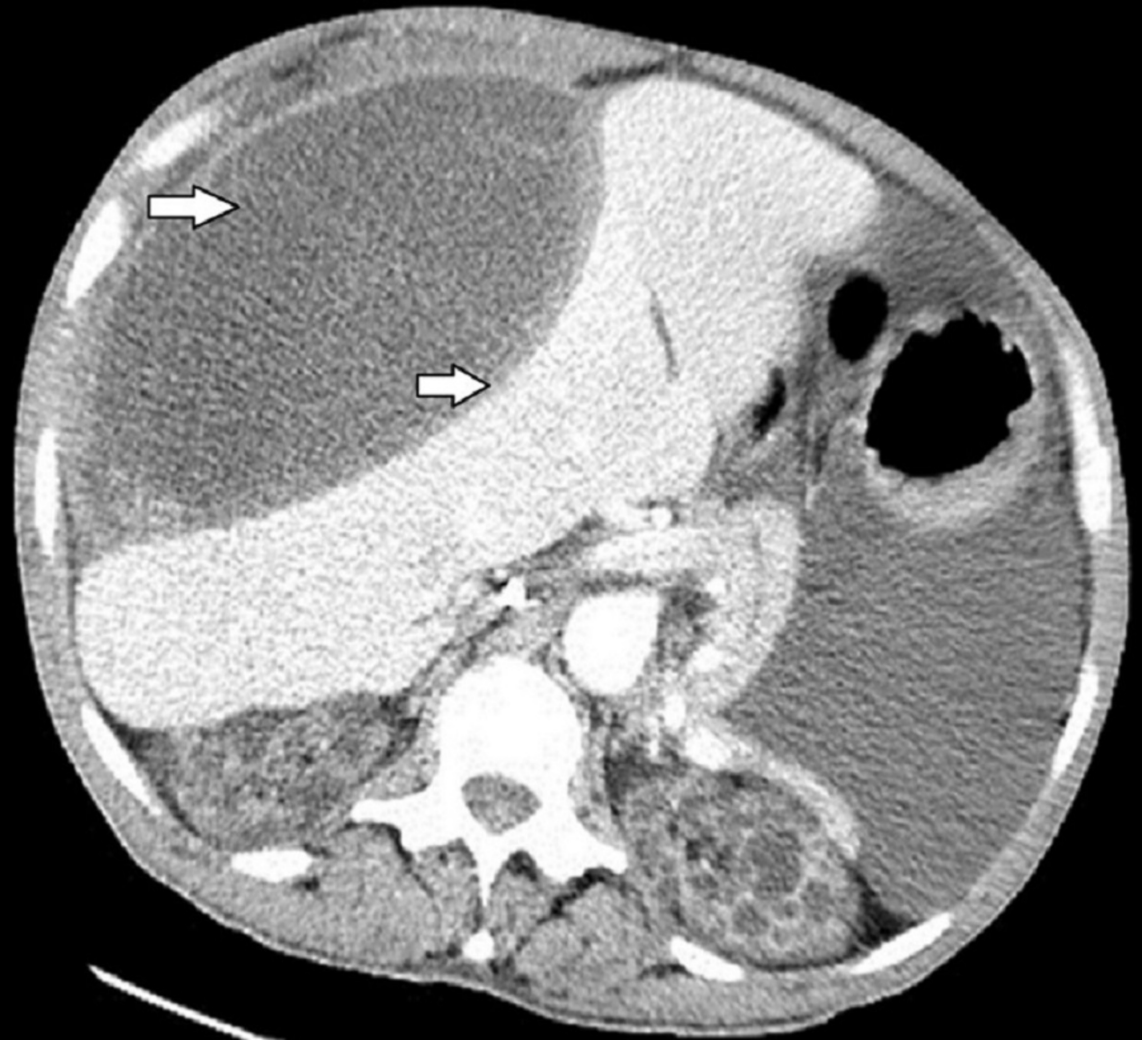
Computed tomography (CT) scan of the abdomen and pelvis revealing encapsulated complex pseudocyst arising from the peritoneal membrane impinging on the liver
